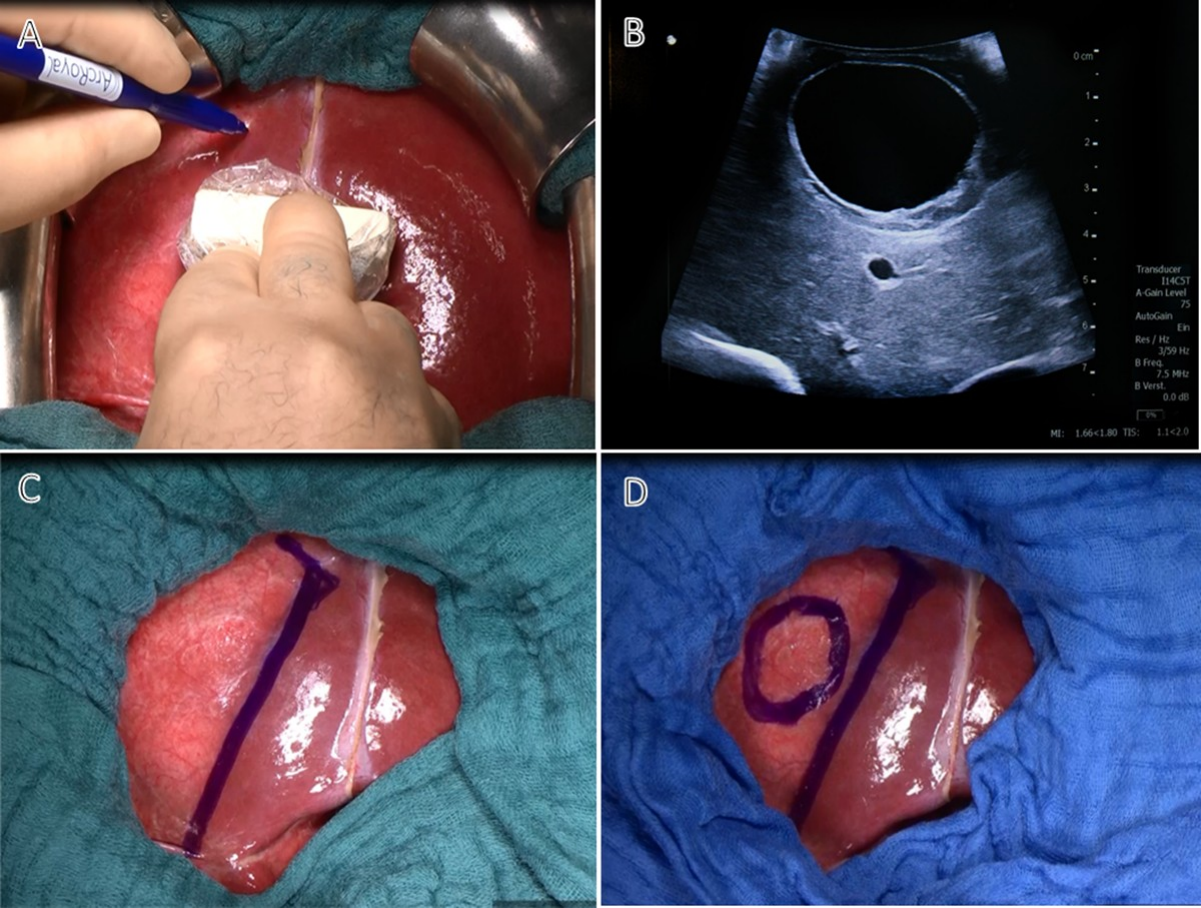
Preparation steps before cyst evacuation. A and B: Cyst localization using ultrasound. C: Protection of the surrounding tissue using sterile green laparotomy sponges (moistened with 0.9% normal saline). D: Second layer of blue laparotomy sponges soaked with 20% sodium chloride.
